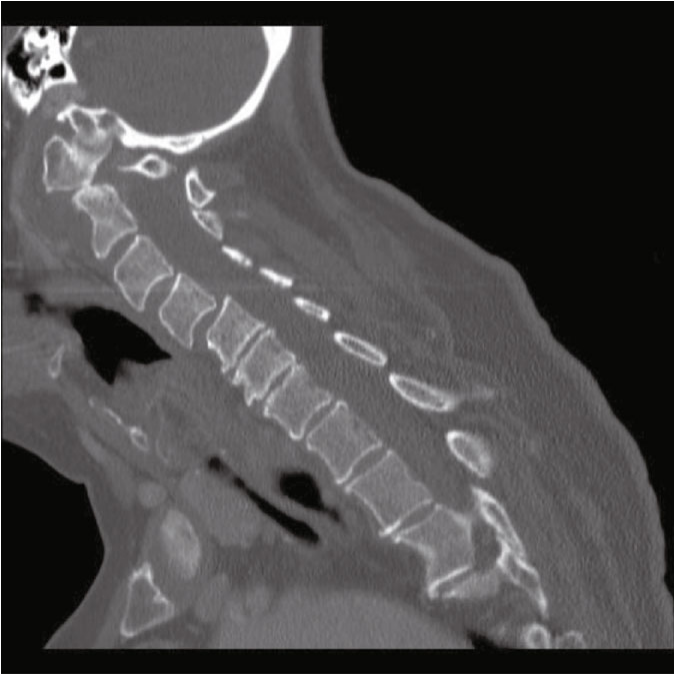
Cervical CT showing subluxation of the posterior C1 ring into the spinal canal.
